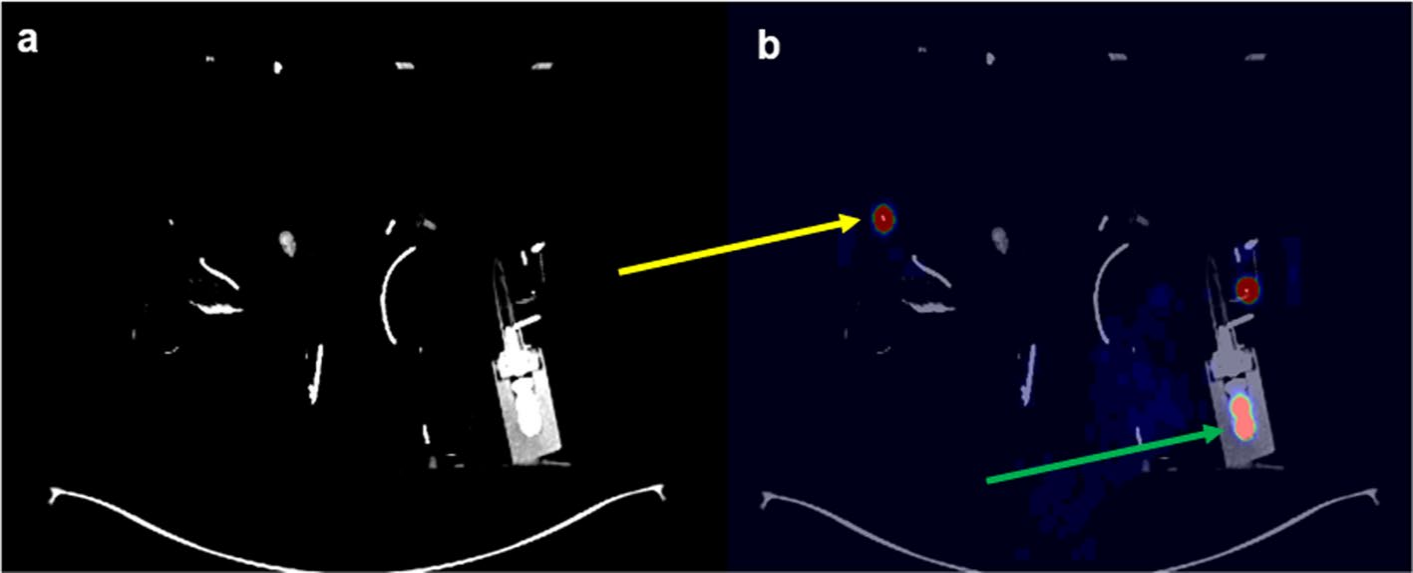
CT (a) and fused ^90^Y-PET/CT images (b) of the ^90^Y-res acquired post-TARE consisting of the administration device including the TheraSphere™ ^90^Y glass microspheres vial (green arrow) and the microcatheter used for the intra-arterial injection during the procedure (orange arrow)
